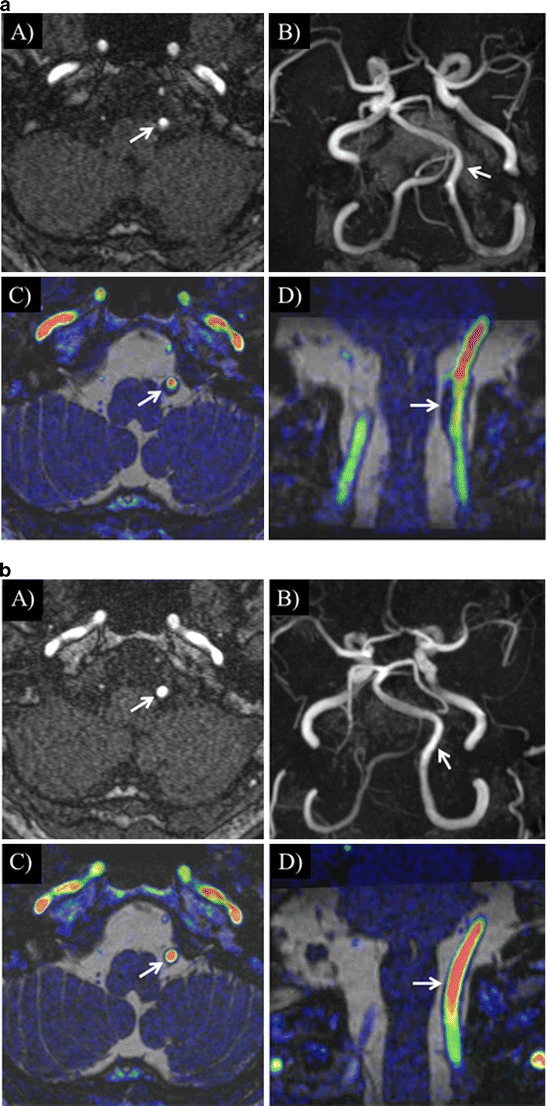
a A 63-year-old man complaining of headache. The left vertebral artery dissection on 3D TOF (*A* and *B*) is not clear (*arrow*). Its shape is not equal to what is evident on fused images. Fused image shows enlargement of the overall vessel diameter with an intramural haematoma in the left vertebral artery. Fused image enabled precise assessment of the relationship between the inner and outer contours of the vessels (*arrow*). (*A*) 3D TOF, (*B*) 3D TOF (MIP), (*C*) TOF and true-FISP fusion (axial), (*D*) TOF and true-FISP fusion (oblique). b Four months later, he underwent follow-up MRI. Vertebral artery dissection was confirmed on imaging follow-up by monitoring normalization of the vessel lumen. Follow-up MRI scan indicated normalization of the vessel lumen (*arrow*). (*A*) 3D TOF, (*B*) 3D TOF (MIP), (*C*) TOF and true-FISP fusion (axial), (*D*) TOF and true-FISP fusion (oblique)
